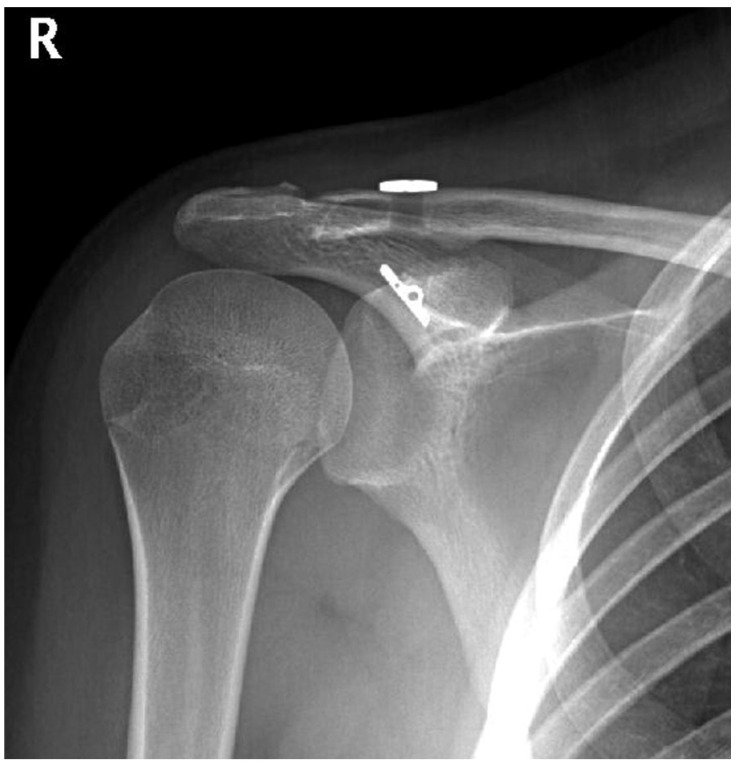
Postoperative radiographic control, with hyper shortening of the acromioclavicular joint.
